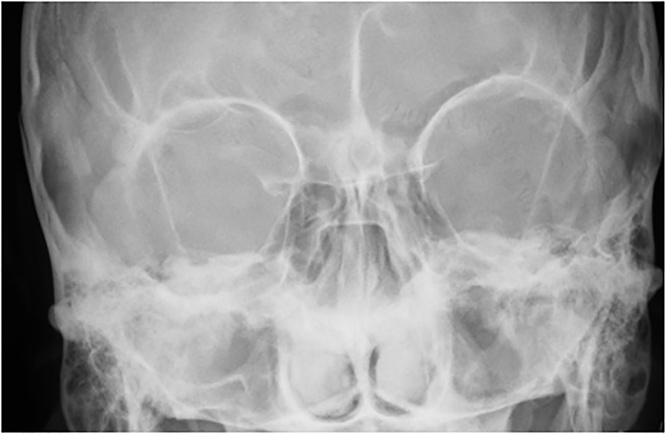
Orbit X-Ray after extraction.
